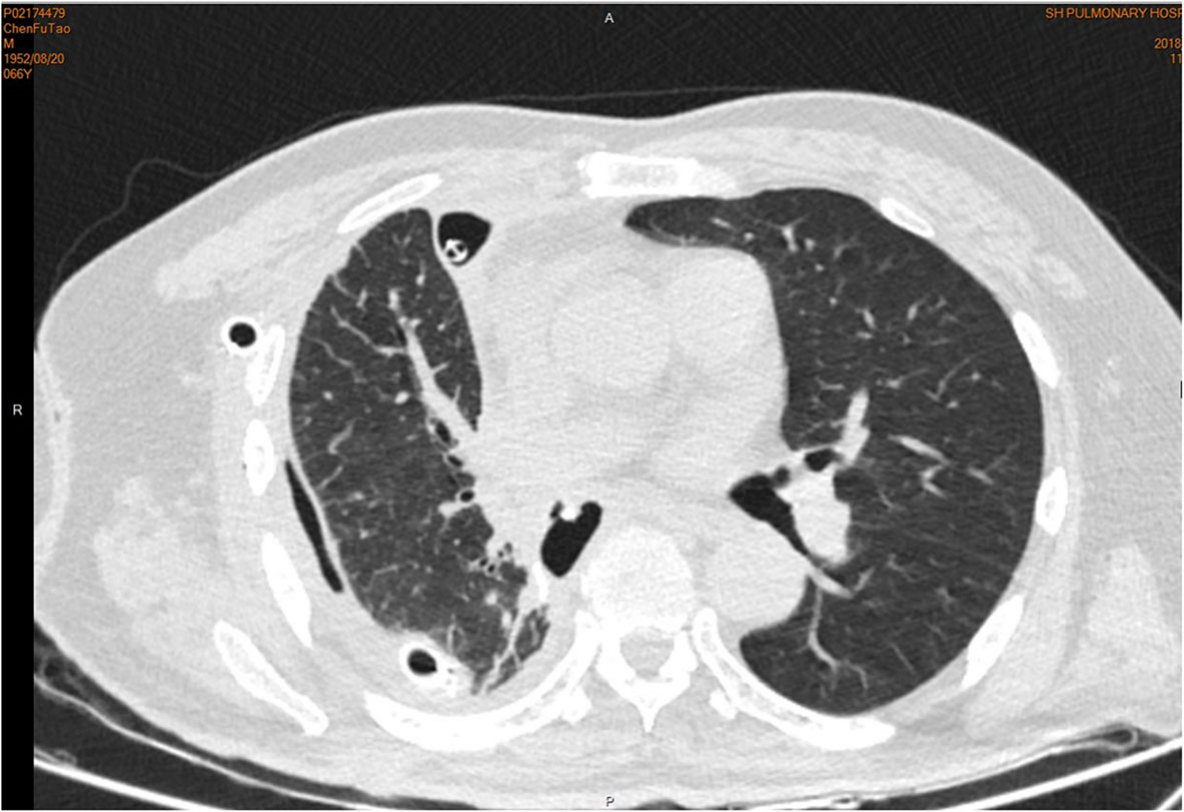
Chest CT scan on the 21th day after second operation. CT scan exhibited BPF on the right intermediate bronchus
